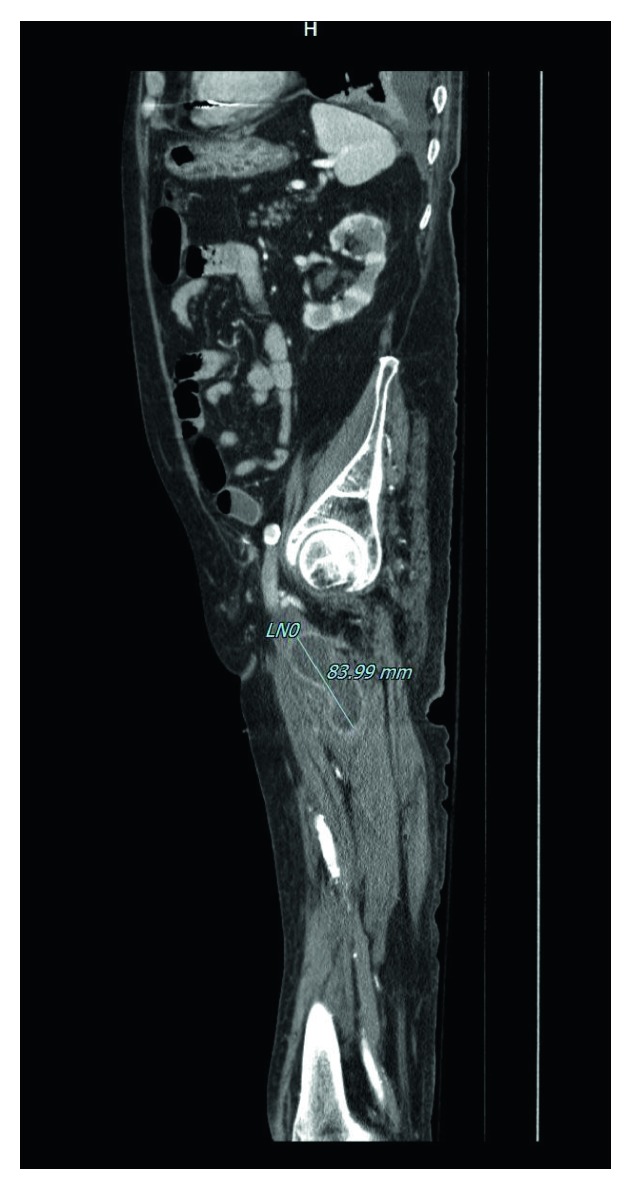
CT scan sagittal section showing the collection still present in the left thigh of the patient after the surgical procedure on the right side.
